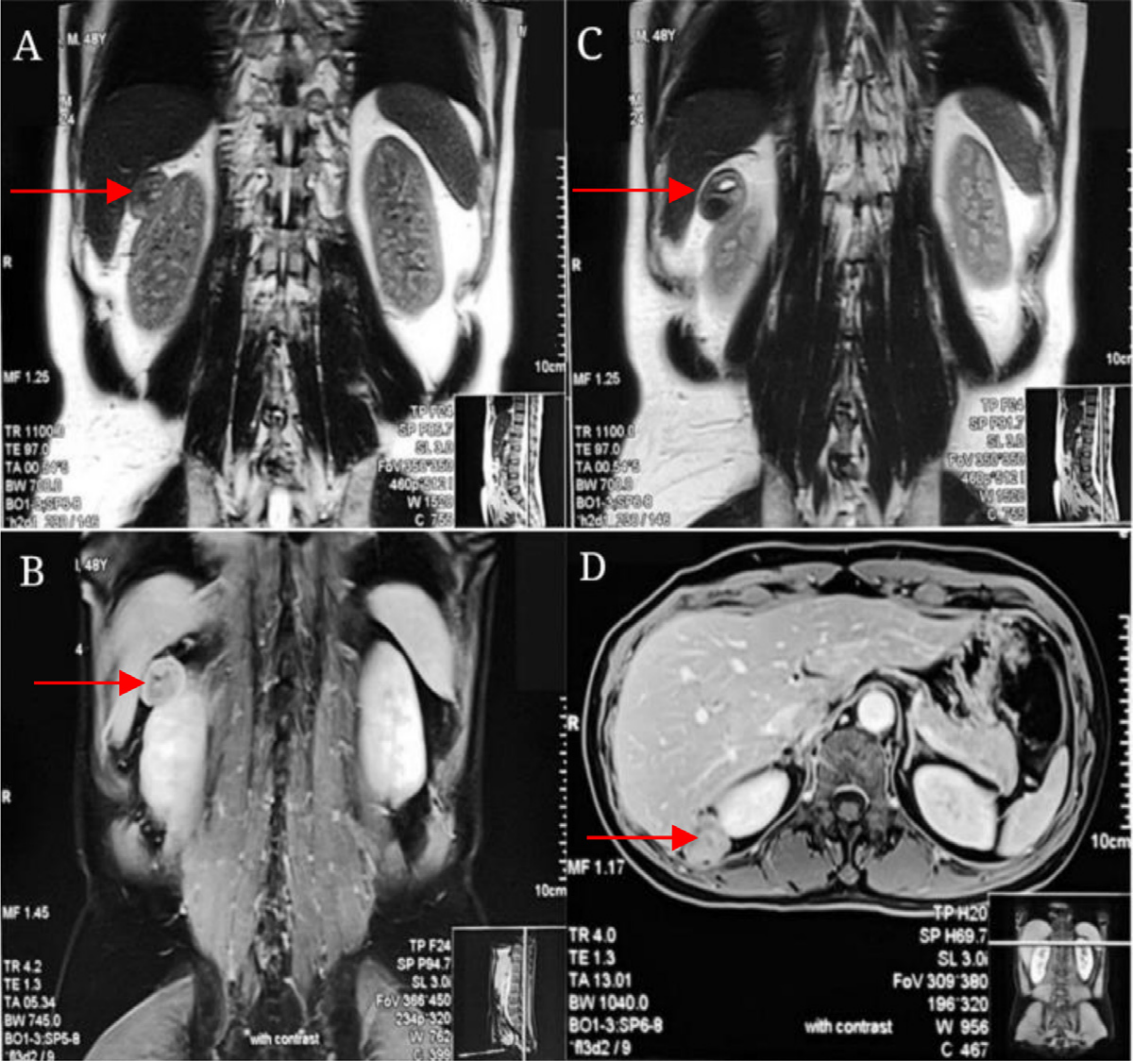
Display of the results of the MRI Abdomen examination with contrast from Dr. Soetomo Academic General Hospital: multiple solid masses measuring 2.9 × 2.7 × 2.9 cm, 1.8 × 2.7 × 2.9 cm, and 4.3 × 1.7 × 4.0 cm in the right adrenal gland.
